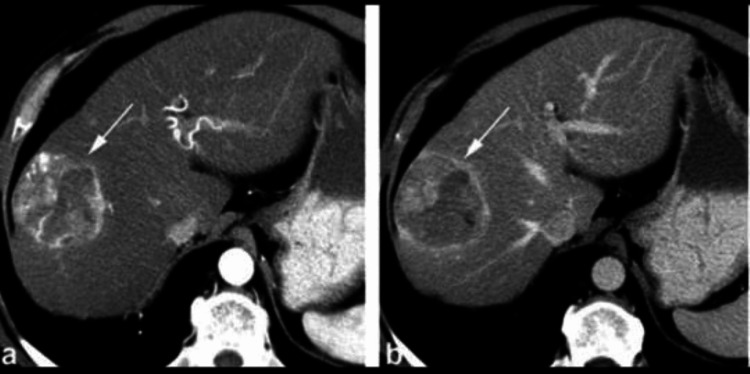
Radiograph showing primary liver lesion
